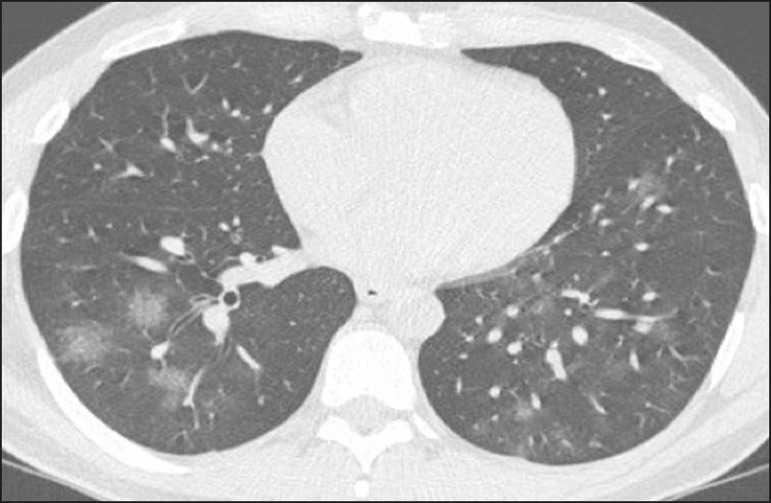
Typical viral pneumonia pattern for on high-resolution CT of the chest, with bilateral ground-glass opacities.Undetermined viral pneumonia pattern (Figure 11)-absence of typical findings and presence of ground-glass opacities with a unilateral, central, tenuous, diffuse, or atypical distributionFigure 11 f11:
